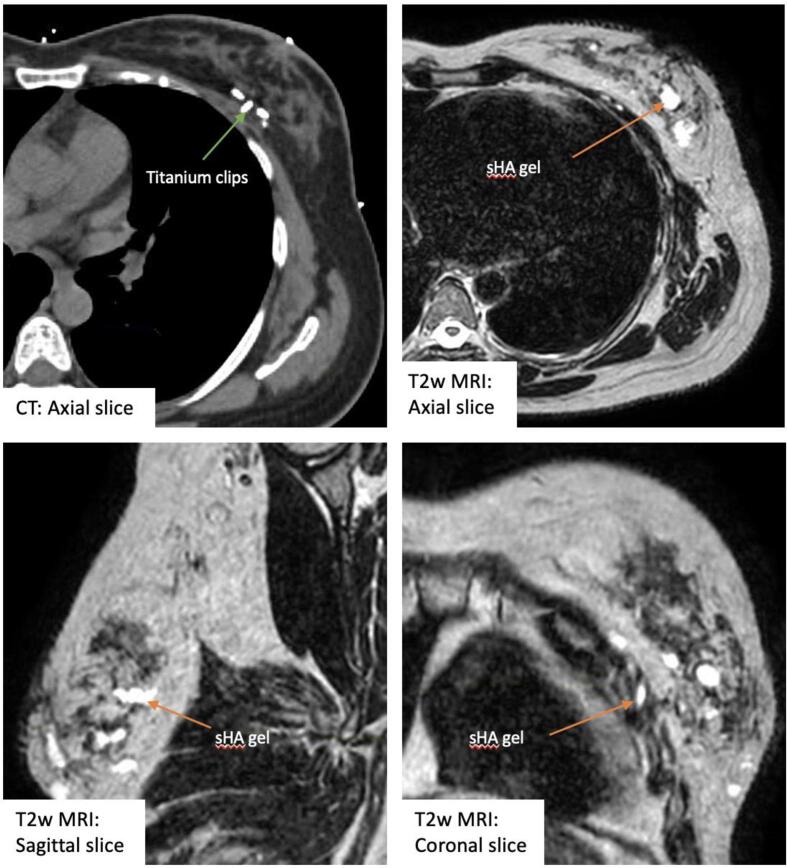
Four-week post-operative computed tomography (CT) and T2-weighted (T2w) magnetic resonance imaging (MRI) scans of a patient with both surgical clips and stabilised hyaluronic acid gel (sHA) gel in-situ.
